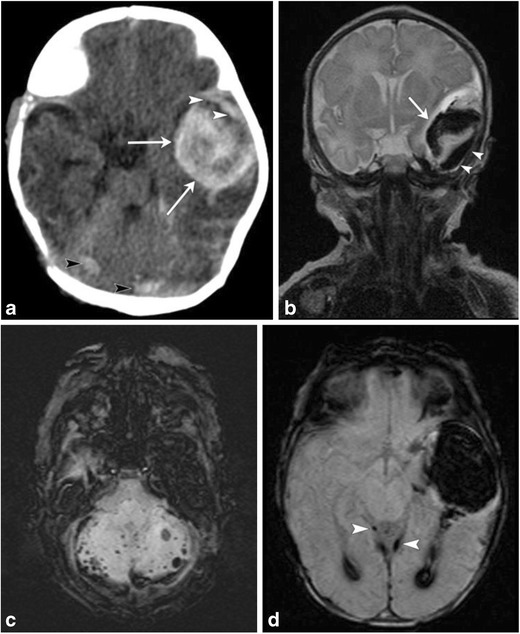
Extraaxial, intraventricular and parenchymal hemorrhages in 3-day-old female post-complicated vaginal delivery presenting with seizures Axial non-contrast head CT (a) and coronal T2 MR image (b) show a large left temporal parenchymal hemorrhage (arrows), with an overlying small subdural hemorrhage (white arrowheads). Small foci of subarachnoid hemorrhage noted along the posterior fossa (white arrowheads on a), with susceptibility artifact on axial susceptibility-weighted image (SWI) (c). Axial SWI MR image (d) through the left temporal hemorrhage also demonstrates blood products within occipital horns of bilateral lateral ventricles and trace subdural hemorrhage along the tentorium (white arrowheads). Follow-up MR at 3 months (not shown) did not demonstrate an underlying left temporal parenchymal mass or vascular malformation
